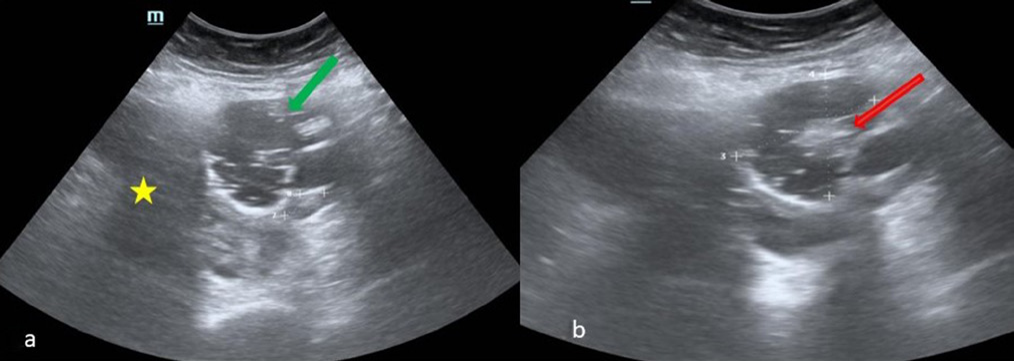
(a, b): Transabdominal ultrasound shows the dermoid component
